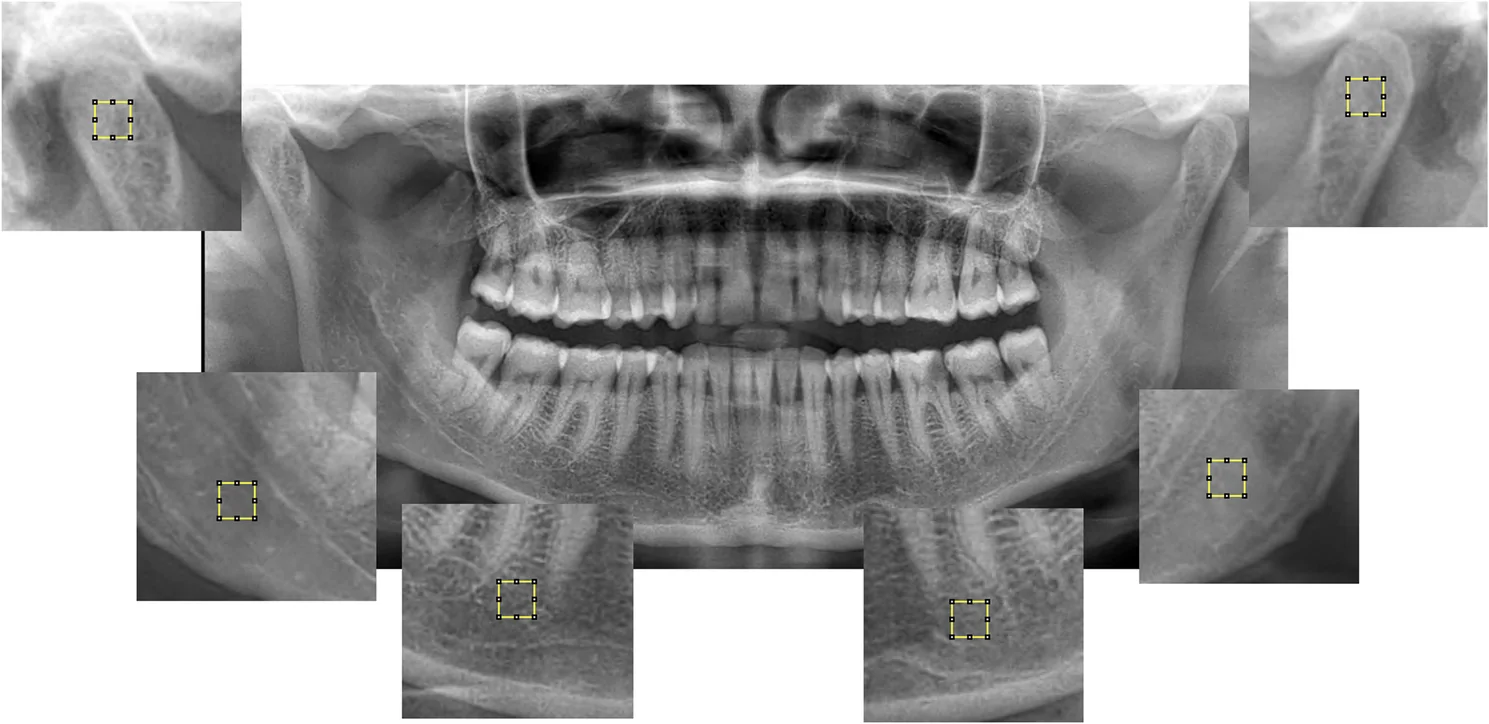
Selection of regions of interest (ROI) on panoramic radiographs. ROI 1: 60 × 60 pixel square at the mandibular condyle; ROI 2: 60 × 60 pixel square located inferior to the mandibular canal in the mandibular angle region; ROI 3: 60 × 60 pixel square located distal to the mental foramen in the mandibular body
